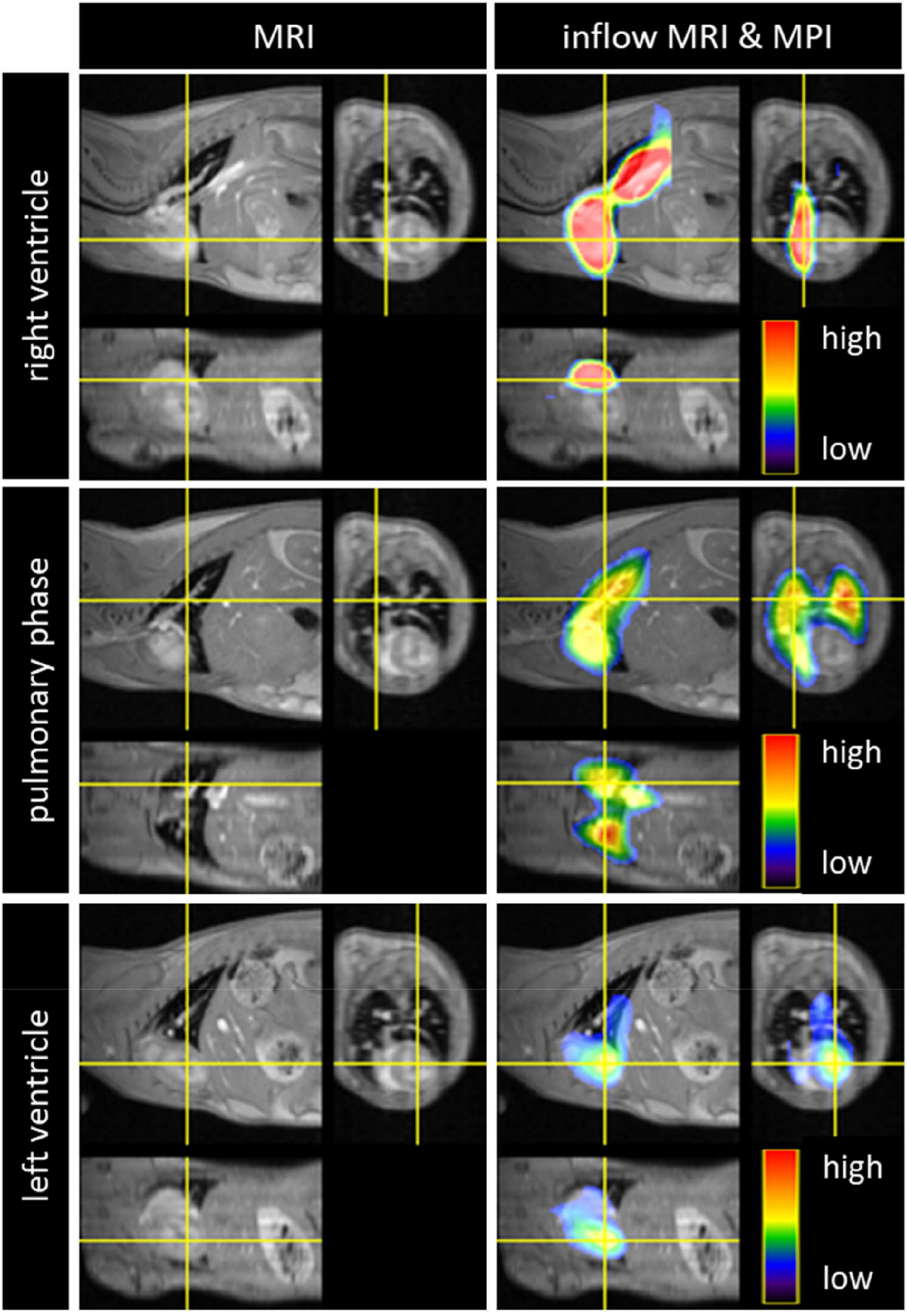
MRI and fused MPI-MRI-data at three distinct time points showing the inflow of tracer into the right ventricle, the passage through the lungs and the arrival at the left ventricle. The MPI signal intensities are color coded. A high amount of tracer per pixel is indicated in red while a low amount in blue.
